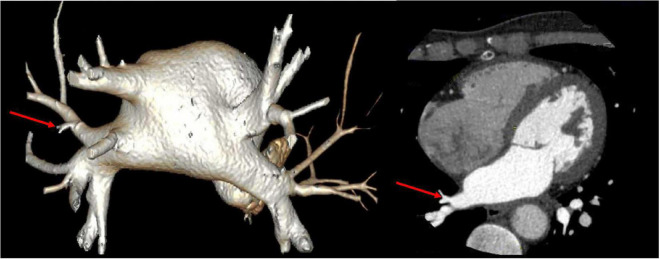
CT angiography (CTA) of left atria-pulmonary vein showed the middle branch of the right pulmonary vein was variable.
